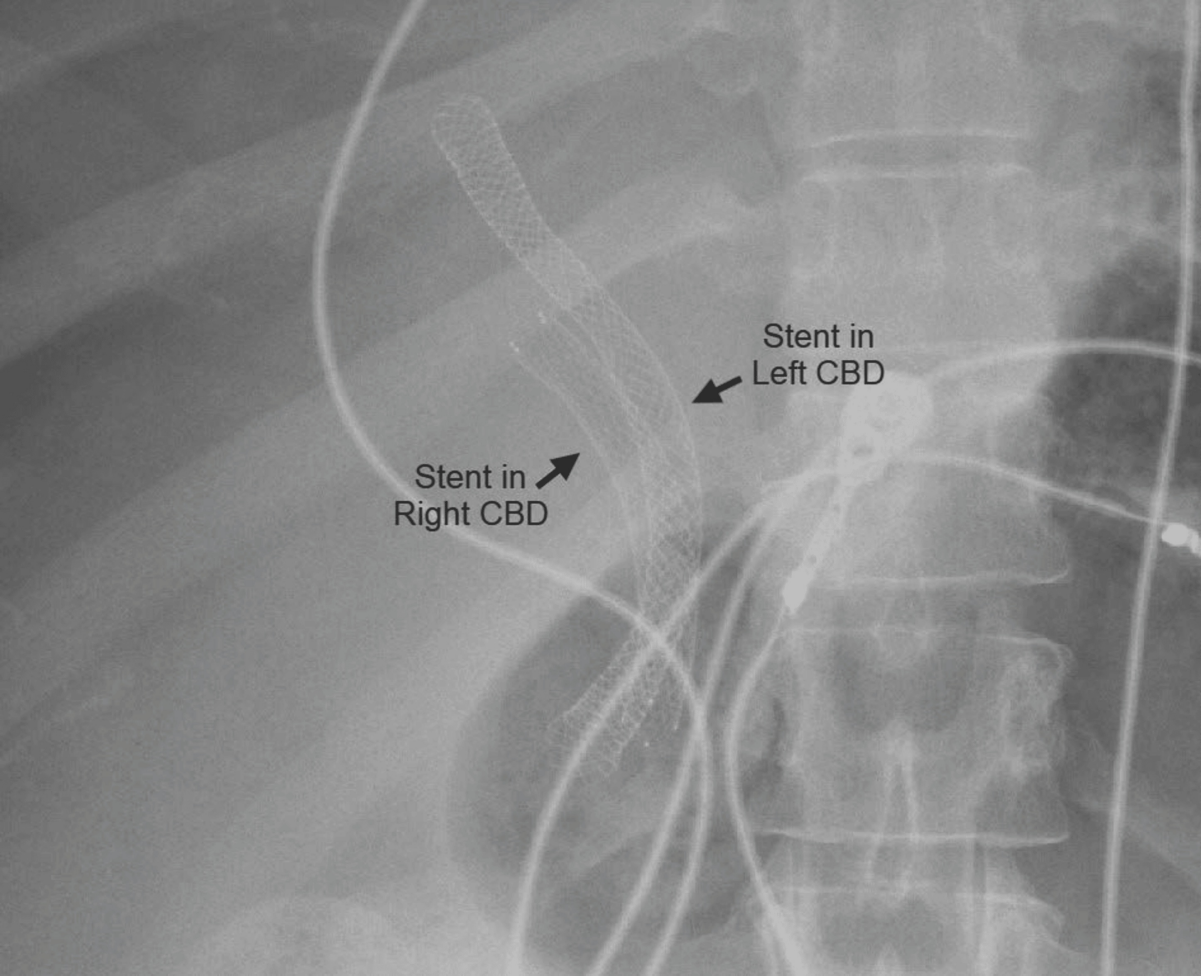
Abdominal radiograph showing the presence of the endoscopically placed metal stent in the left CBD and the IR-placed metal stent in the right CBD (both metal stents can be seen exiting the biliary system through the major papilla) CBD, common bile duct; IR, interventional radiology
